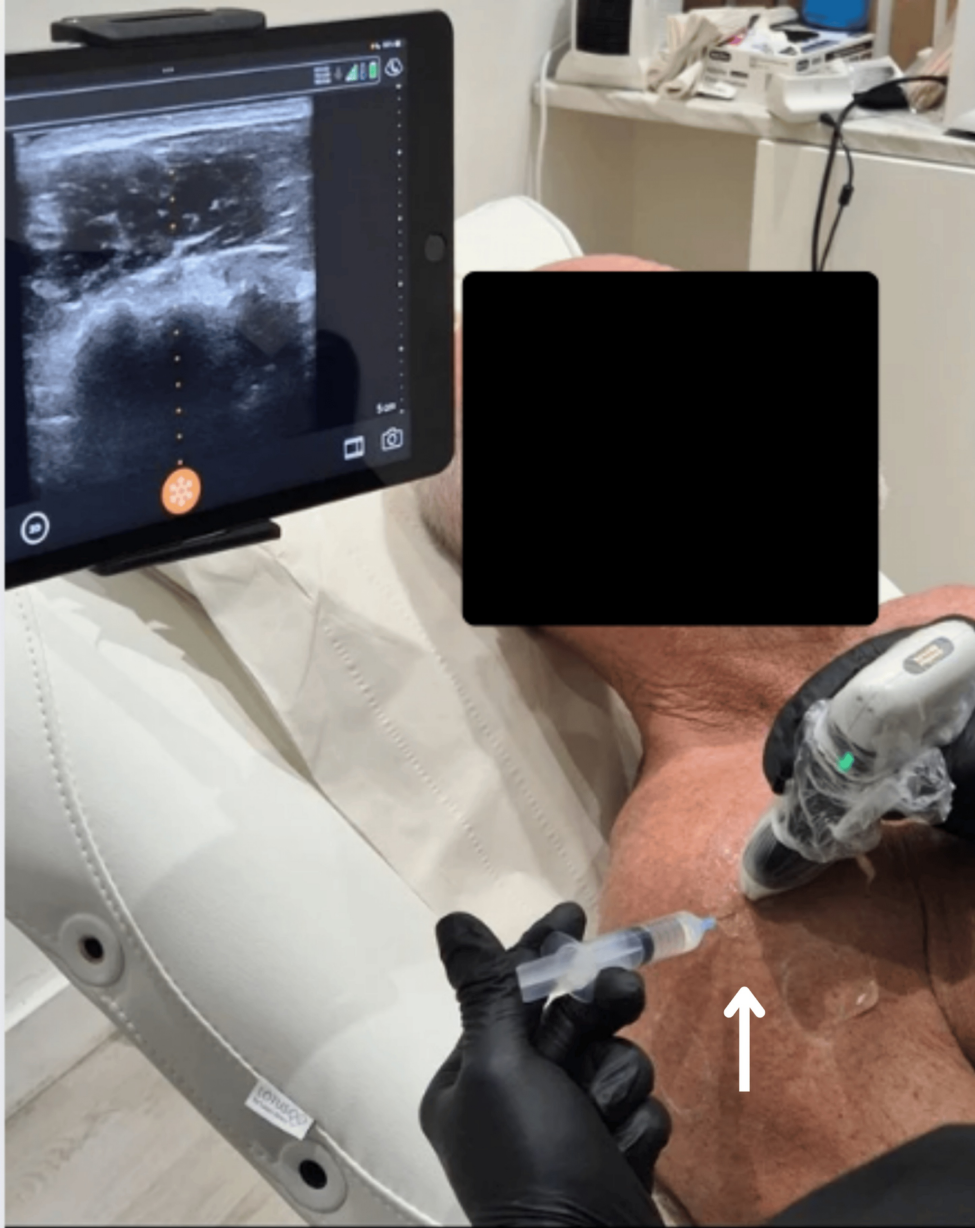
Administration of local anesthesia under ultrasound guidance (white arrow) post skin preparation
